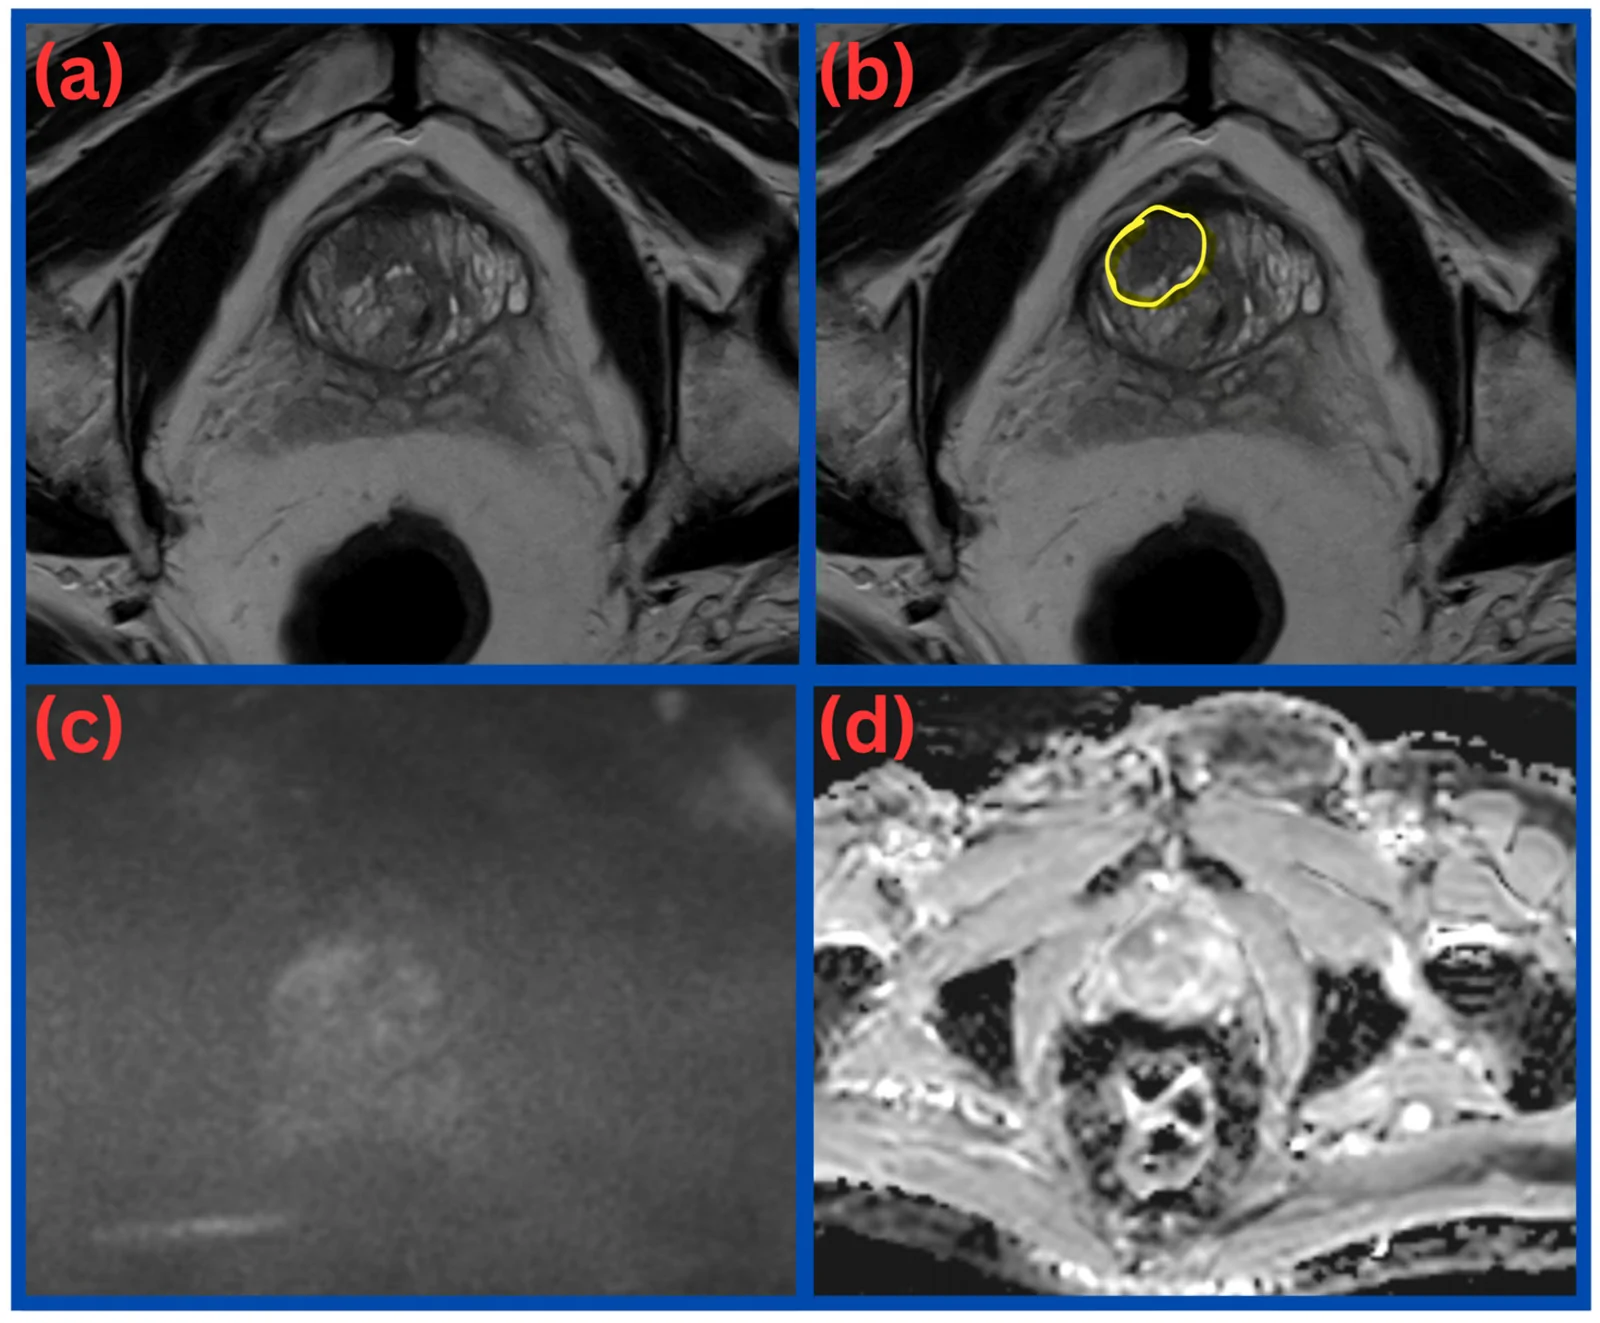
Multiparametric MRI of a PI-RADS 3 lesion. (a) Axial T2-weighted image showing a reasonably well-delimited area of T2 hypointensity in the antero-lateral right transition zone, and (b) the corresponding axial T2-weighted image with the lesion outlined in yellow. (c) High *b*-value diffusion-weighted image (*b* = 1400 s/mm^2^) shows only minimal high signal, and (d) the ADC map shows only mild corresponding hypointensity, indicating equivocal (mild) diffusion restriction. The intermediate appearance—T2 hypointensity with only mild diffusion correlate—is characteristic of a PI-RADS 3 (intermediate) assessment, the category for which the decision to biopsy or to use ancillary risk stratification such as PSA density is most finely balanced.
